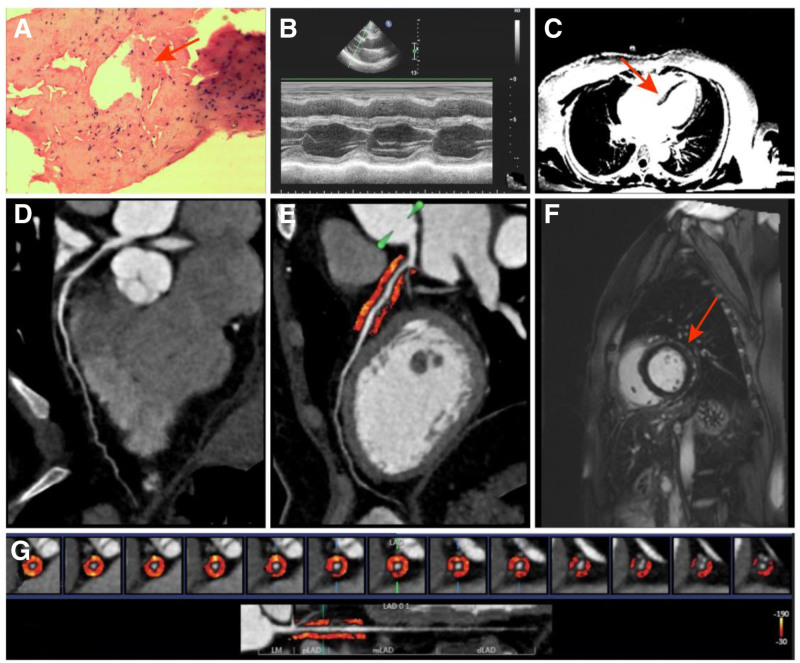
(A) The pathological section of thrombus. (B) The ventricular wall motion in cardiac ultrasound. (C and F) The myocardial magnetic resonance imaging results. (D, E, and G) The CTA and FAI results of the patient’s LAD. CTA = computed tomography angiogram, dLAD = distal LAD, FAI = fat attenuation index, LAD = left anterior descending, LM = left main lesion, mLAD = middle LAD, pLAD = proximal LAD.
